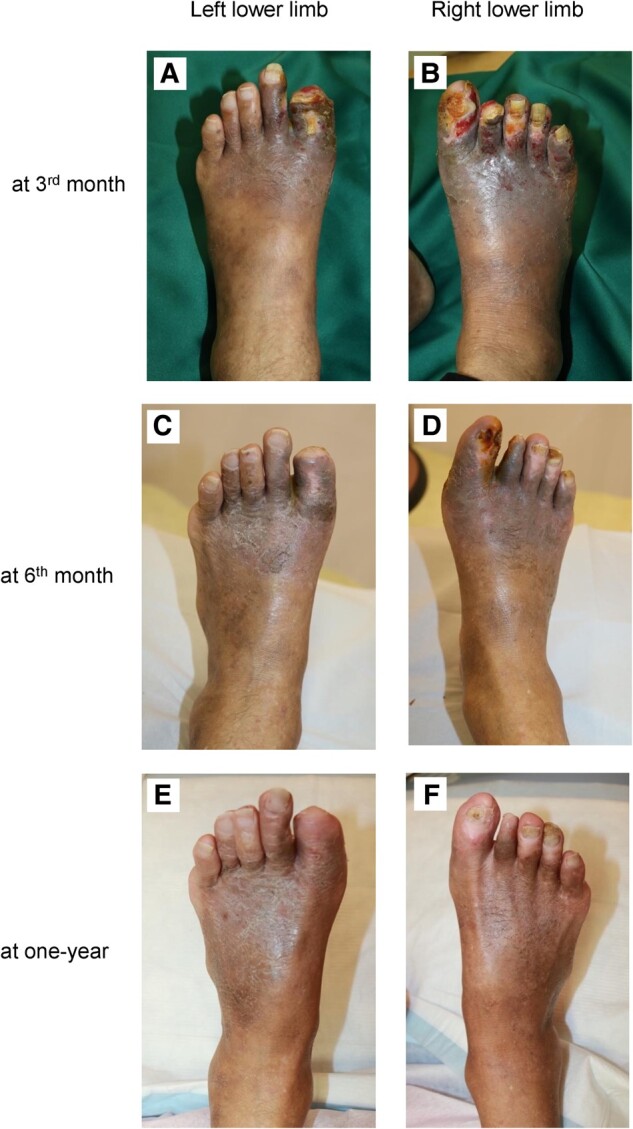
Images of the patient’s feet during ongoing treatment with low-intensity pulsed ultrasound at the 3rd month (*A*, left limb and *B*, right limb) with a visual analogue scale of 75, at the 6th month (*C*, left limb and *D*, right limb) with a visual analogue scale of 40, and at 1 year (*E*, left limb and *F*, right limb) with a visual analogue scale of 0.
